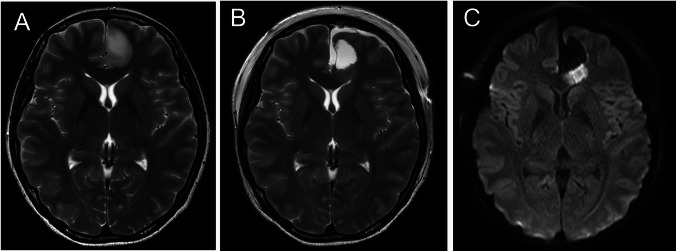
Typical ischemic lesion after cingulate gyrus resection without injury to the corpus callosum. A 24-year-old woman underwent MRI for evaluation of headaches. An enlarged T2 high-signal area was observed in the anterior cingulate gyrus (A). A low-grade glioma was suspected, and total resection was performed without injury to the corpus callosum (B). Postoperative MRI showed an ischemic area in the corpus callosum adjacent to the resected area (C). Postoperatively, the patient exhibited no new neurologic symptoms
